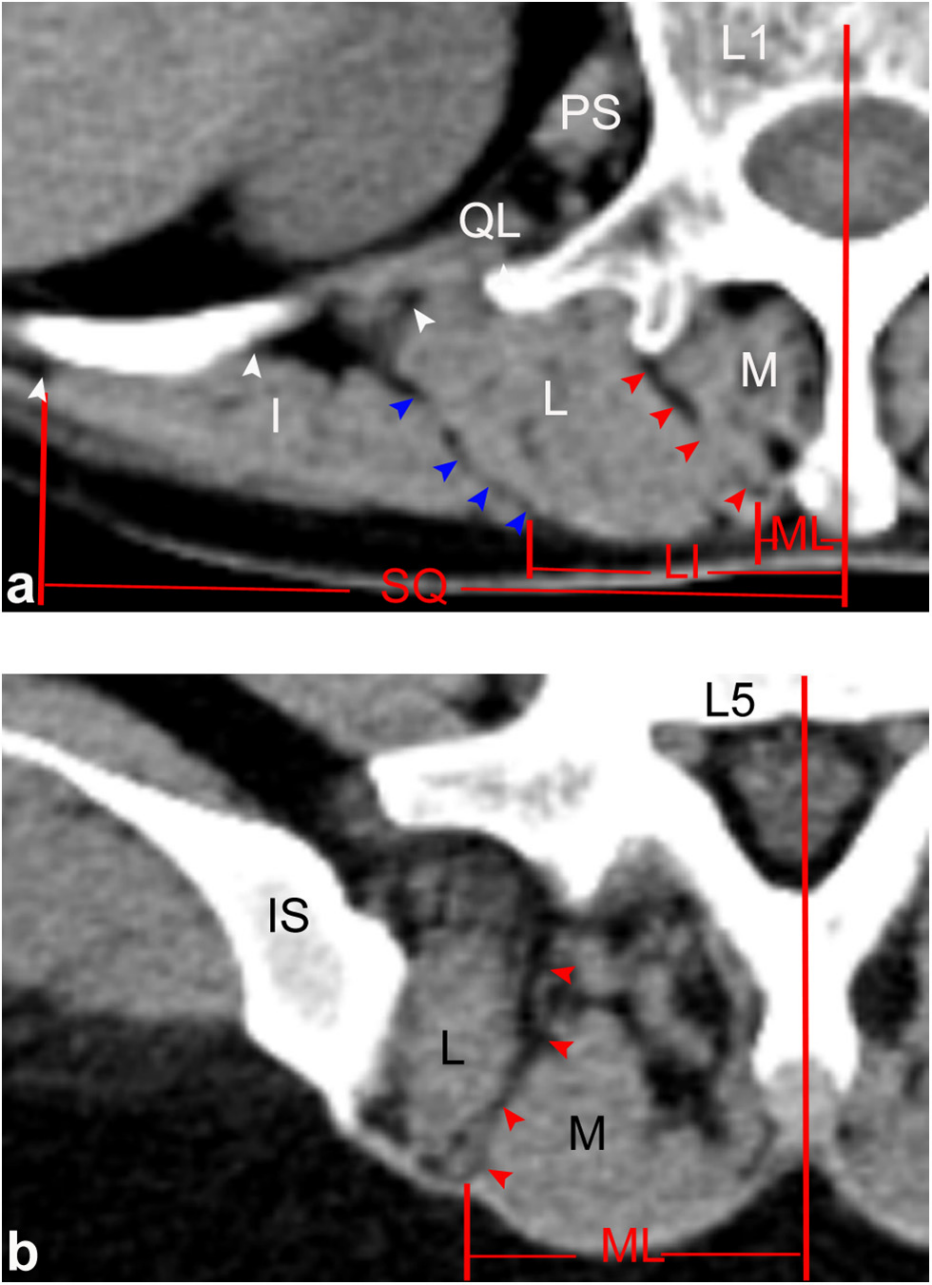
Horizontal views of the posterior lumbar spine in CT images. (a) Through the upper level of the lumbar spine (L1), ML (red arrows), SQ (white arrows) and LI (blue arrows) were identified by line-shaped low densities within the paraspinal muscles. (b) Through the lower level of the lumbar spine (L5), only the ML was identified. *M*: multifidus; *L*: longissimus; *I*: iliocostalis; *IS*: iliac spine; *QL*: quadratus lumborum; *PS*: psoas major.
